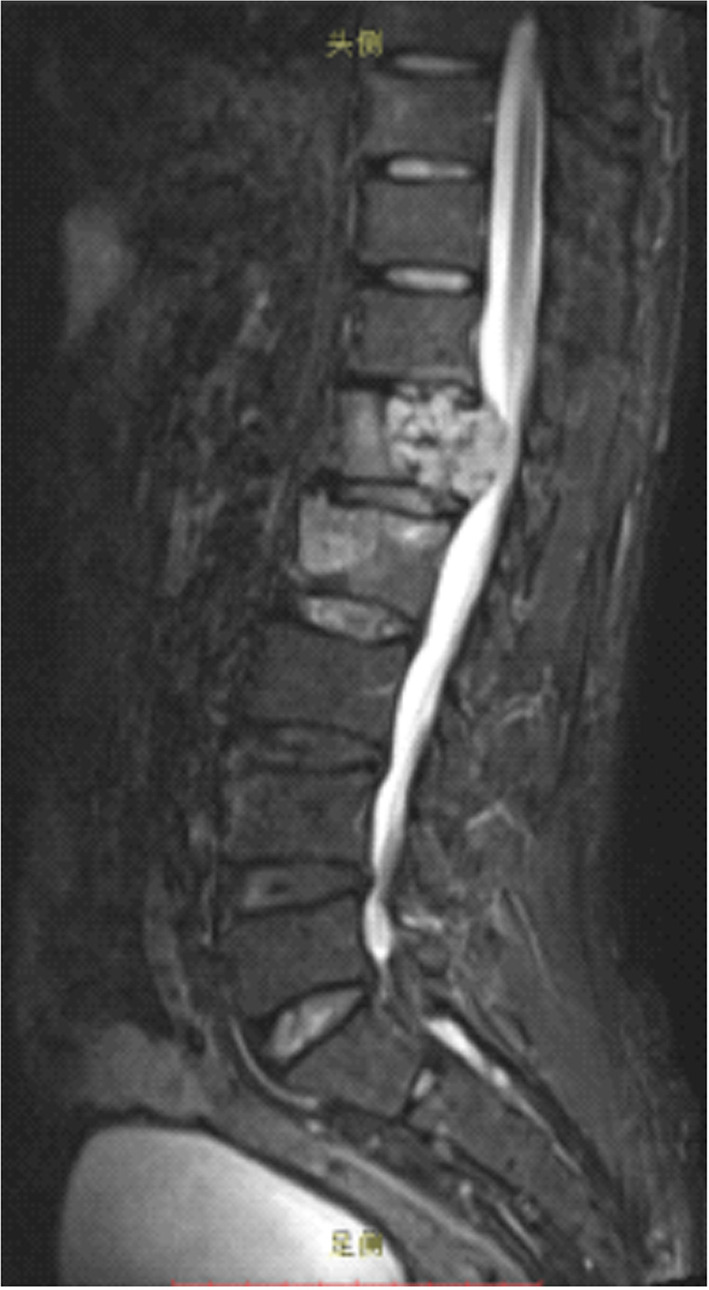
T2 sagittal MRI of the spine in a 57‐year‐old male, it showed lesions with signal abnormalities in L1 and L2 vertebrae, lumbar spinal canal stenosis with cord compression caused by extended lesion at L1 level.
